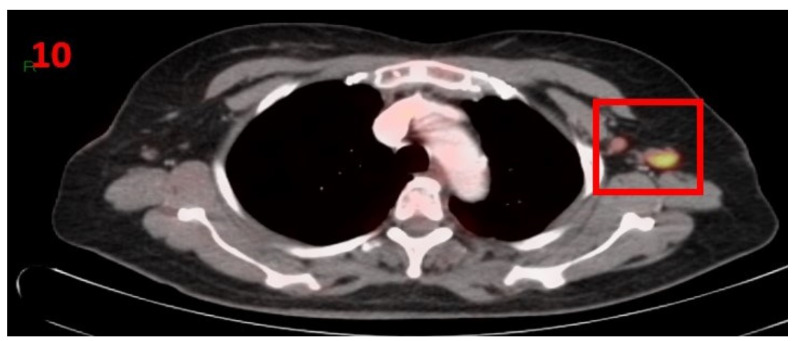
[18F]FDG PET-CT showing hypermetabolic uptake in the 10th patient with lymphadenopathy in the left axillary region (red square).
